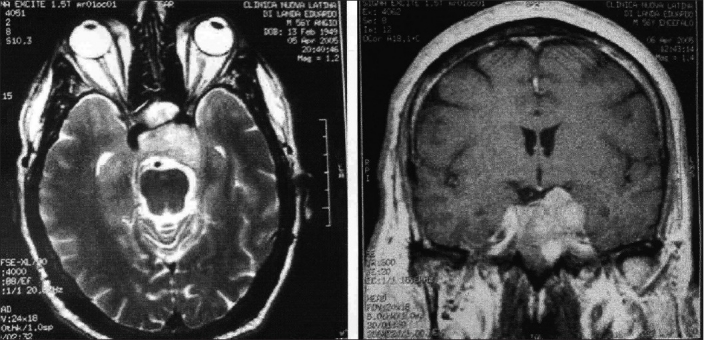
a) MRI scan, axial T2 image. A pituitary tumor invading the left CS and encasing the ICA is demonstrated. b) The T1 post-contrast coronal scan gives clear evidence of the extra-sellar lateral and superior extensions of the lesion
